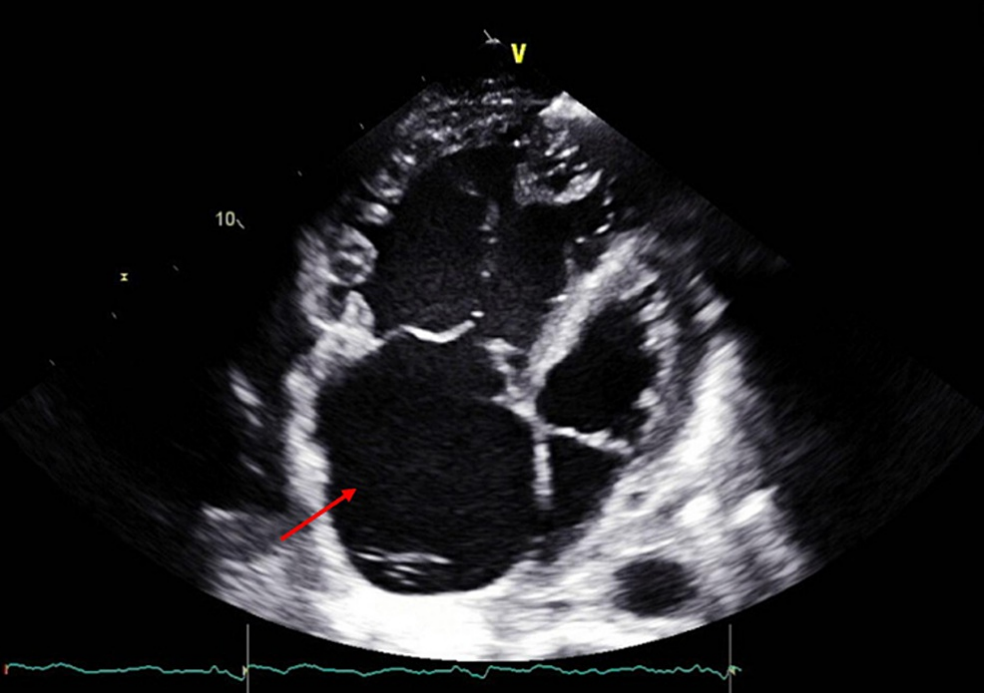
Echocardiogram four-chamber views showing severely dilated right atrium and right ventricle, with no evidence of vegetations.
